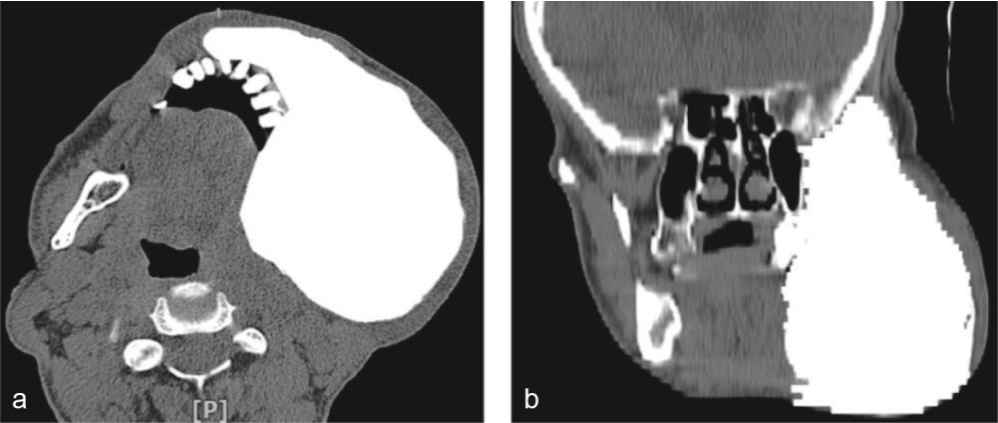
Preoperative computed tomography volume measurement (a: cross-section, b: coronal)
